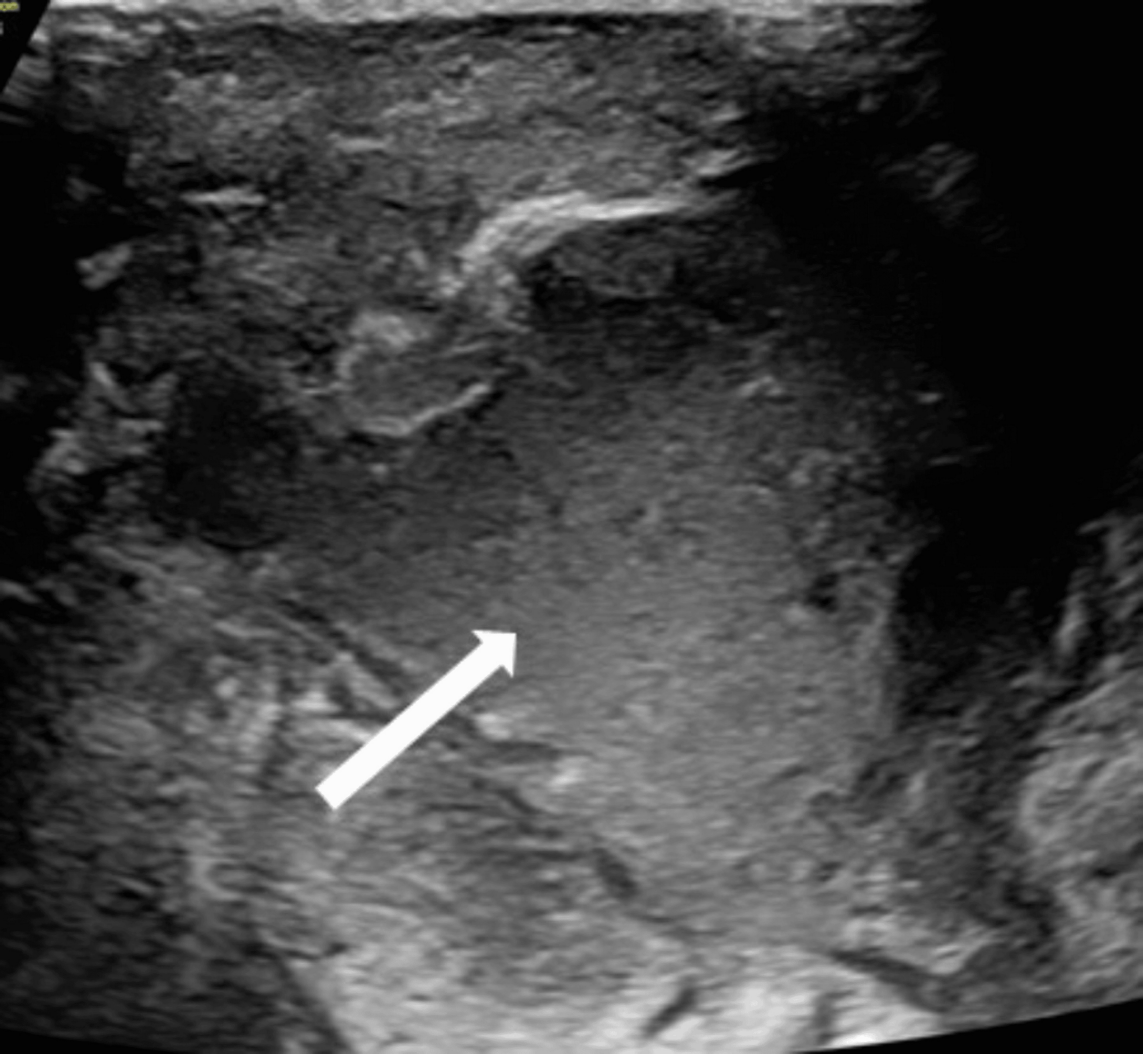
USG of the left scrotum shows hypoechoic collection with Brownian movements noted within the left scrotal sac (long white arrow) Brownian movements: random, erratic movement of small particles suspended in a fluid as they collide with the fast-moving molecules of the surrounding fluid USG, ultrasonogram
